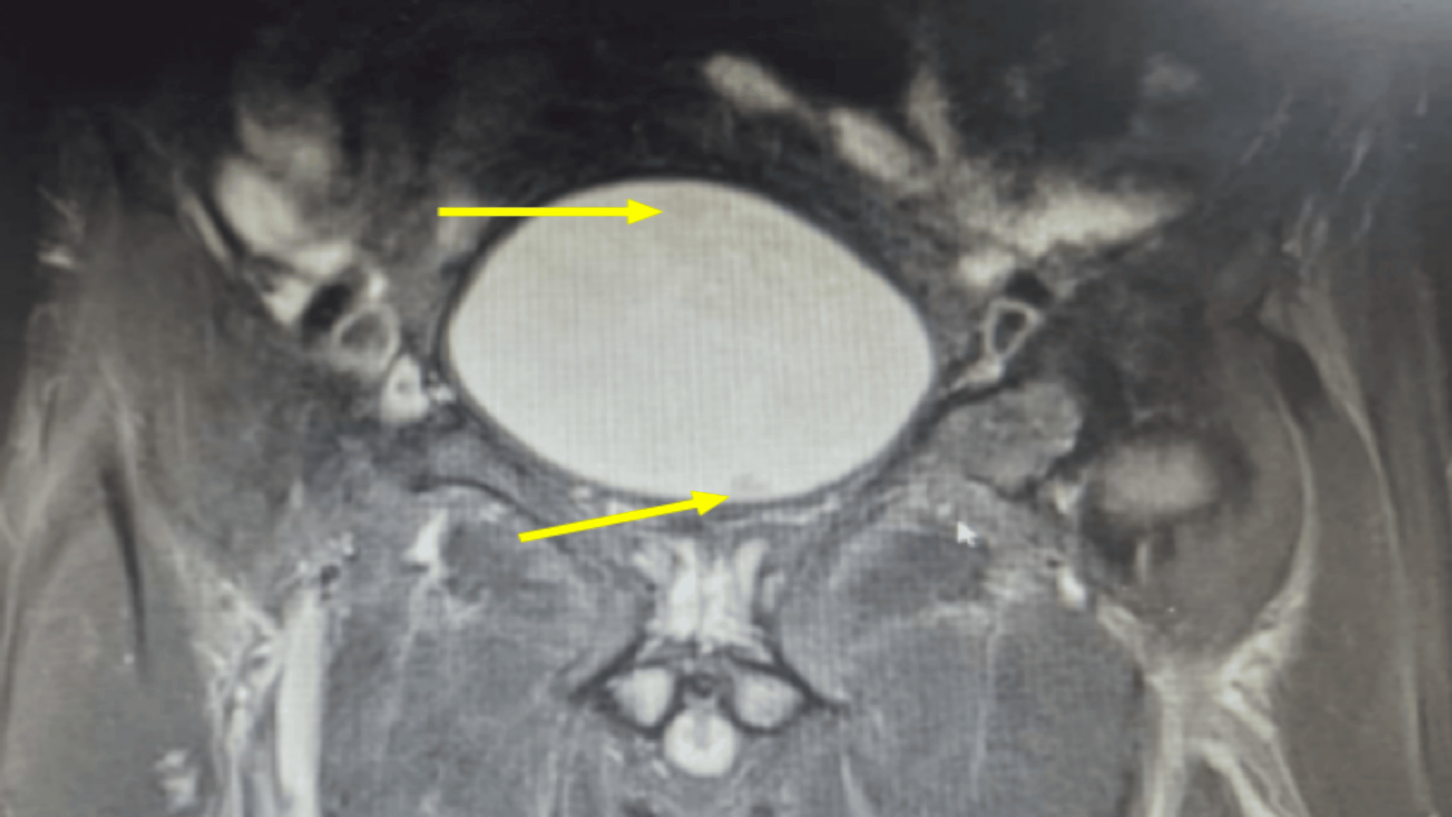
MRI of the pelvis showing polypoidal lesions at the bladder dome and base T2-weighted MRI showing a 3.5 cm lesion arising from the bladder dome (arrow) and a 1.6 cm lesion arising from the bladder base (arrow) MRI: magnetic resonance imaging
